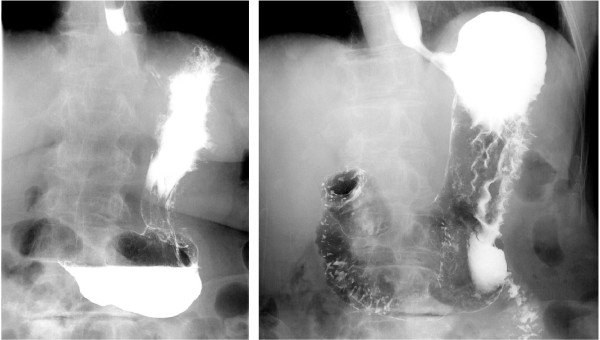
Barium upper gastrointestinal X-ray series findings. Barium upper gastrointestinal X-ray series indicating rigidity and poor extensibility of the gastric wall from the upper to lower gastric body.
